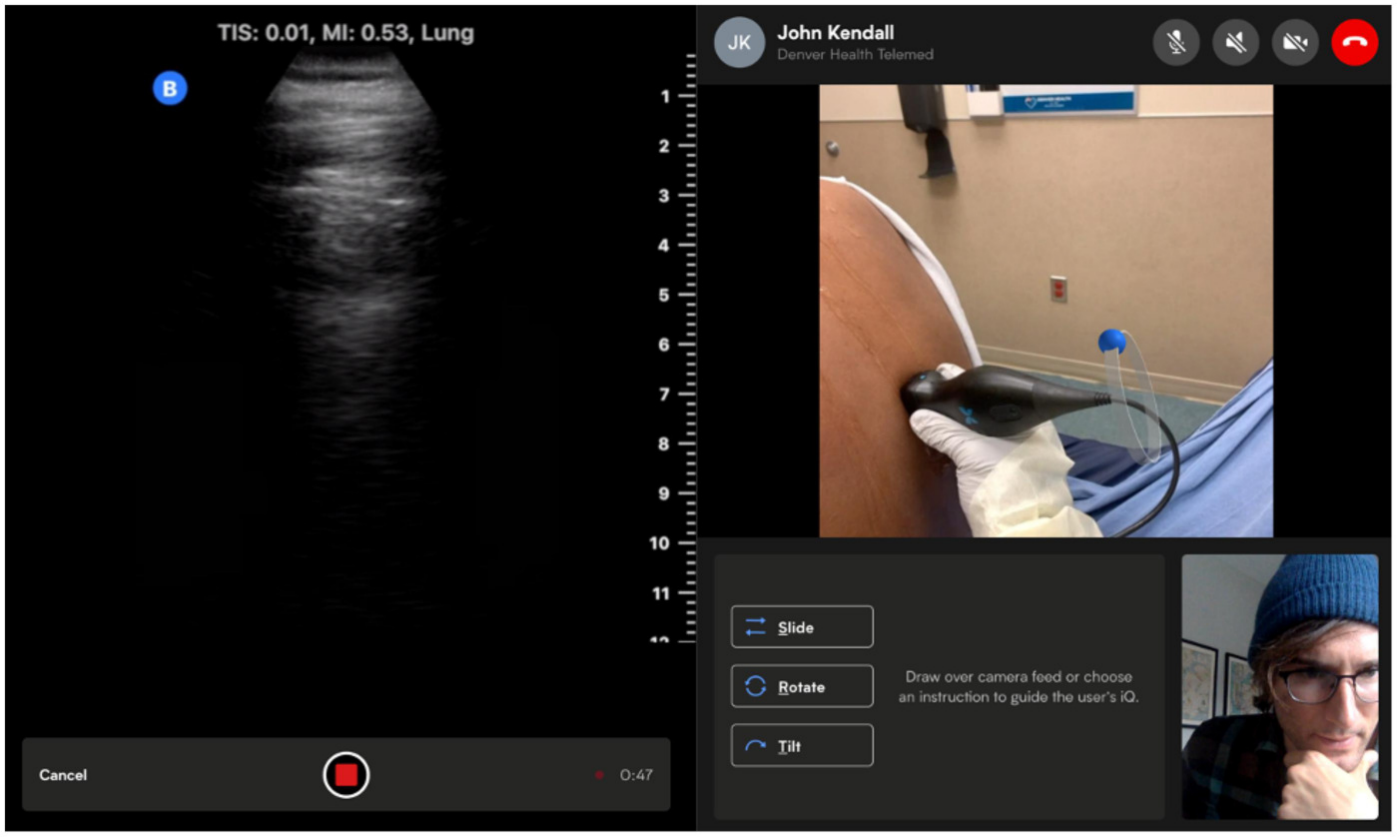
A tele-ultrasound user interface. Figure contains images of the author(s) only.
